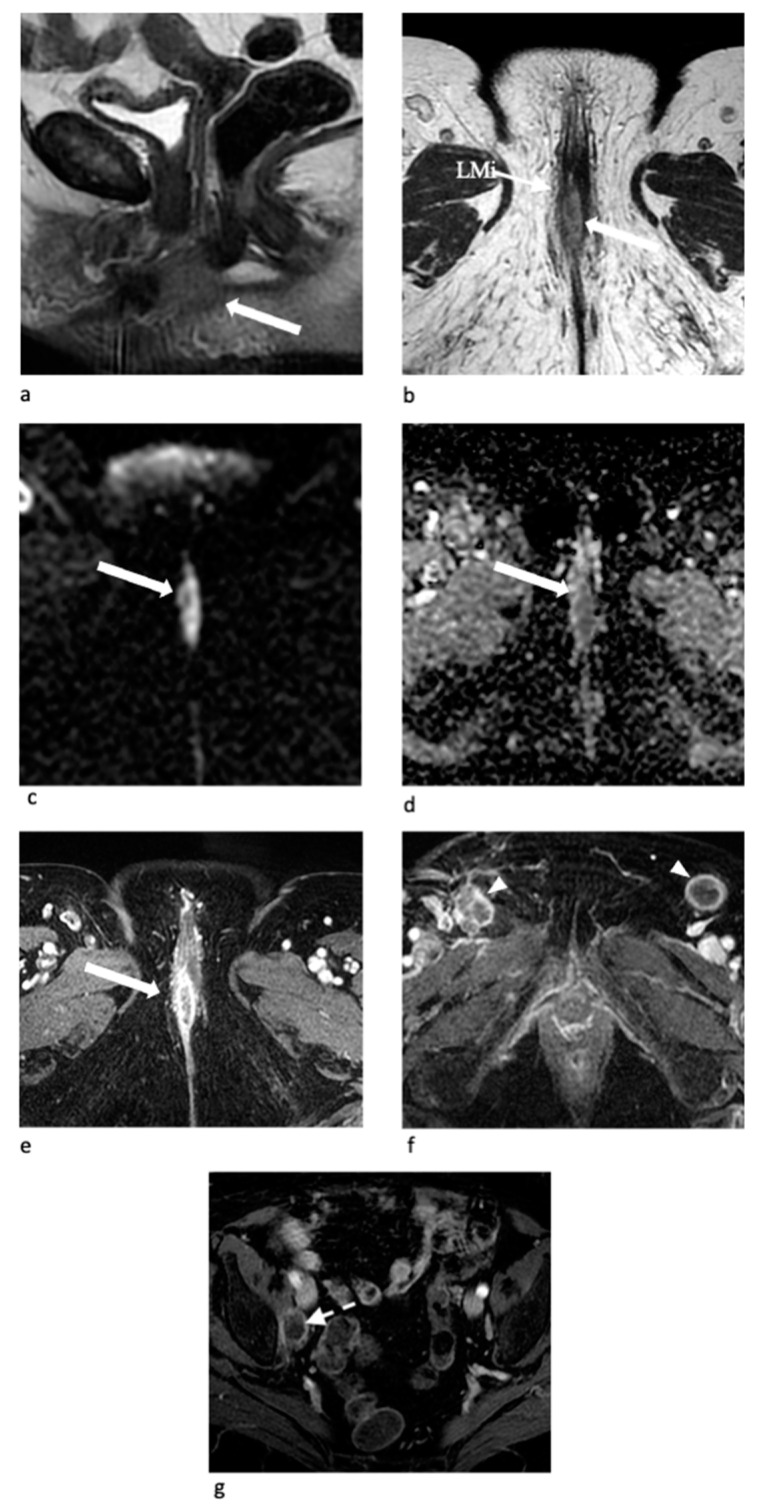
Stage IVB, in a 75-year-old woman with vulvar carcinoma. Sagittal (a) and axial (b) T2-W MR images show a midline vulvar mass (arrow) involving the right labium minus (LMi) with hyperintense signal intensity on axial DWI (c) and marked diffusion restriction on the corresponding ADC map (d). Axial contrast-enhanced T1-W MR images at the level of perineum (e) and of pubic symphysis (f) show early arterial enhancement of the tumor (arrow) and necrotic bilateral inguinal lymph nodes (arrowheads). Axial contrast-enhanced T1-W MR image on the pelvis also shows the presence of an enlarged and necrotic right obturator lymph node (dotted arrow) (g).
